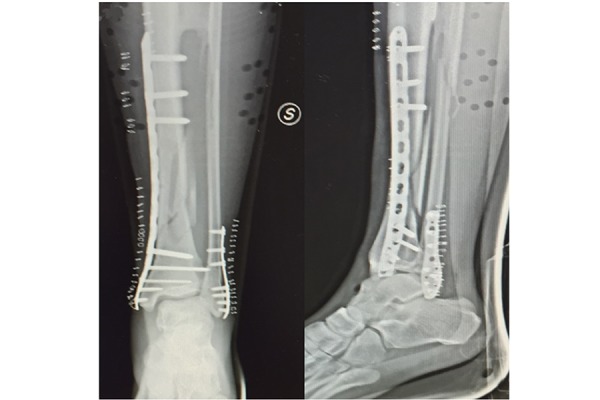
X-ray shows the final osteosynthesis
